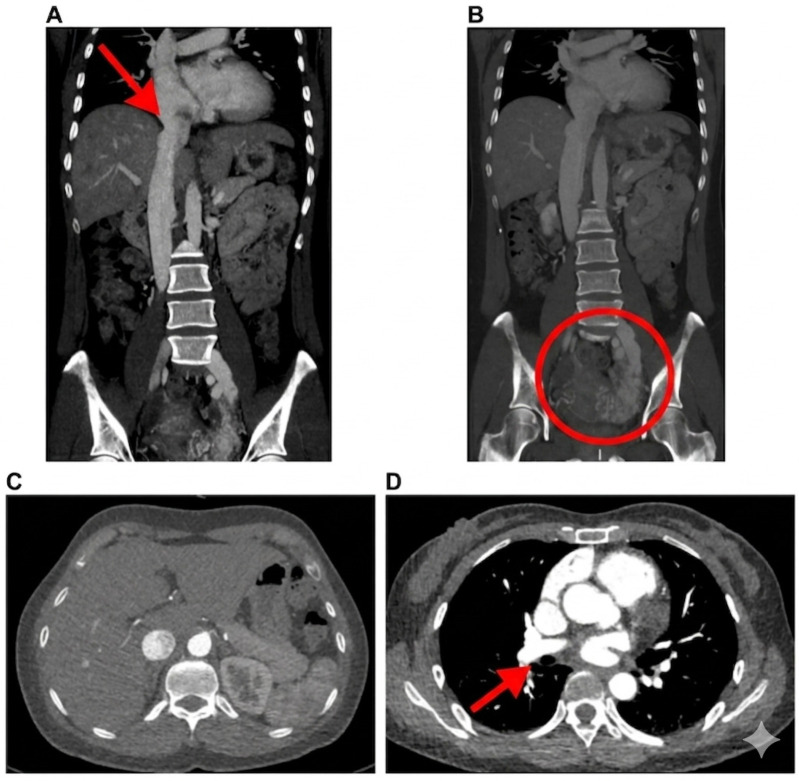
Preoperative inferior vena cava computed tomographic angiography. (A) Inferior vena cava CTA sagittal reconstruction section; (B) Inferior vena cava CTA sagittal reconstruction section (another perspective); (C) Inferior vena cava CTA pelvic axial (cross section) section; (D) Inferior vena cava CTA axial section of the chest.
